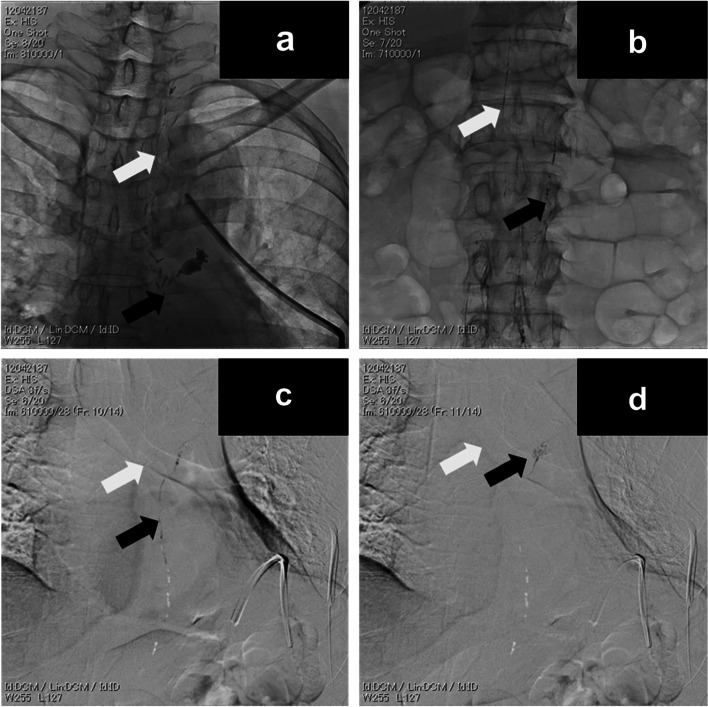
Fluoroscopic X-ray image during lymphangiography. a Two thoracic ducts (black arrow, white arrow) were observed. No connections were observed. b Chyle vessels (black arrow, white arrow) were present in the abdominal cavity. No cisterna chyli was observed. c Thoracic duct (black arrow). Left main bronchus (white arrow). d Leakage site from the thoracic duct running through the left thoracic cavity (black arrow). Left main bronchus (white arrow)
